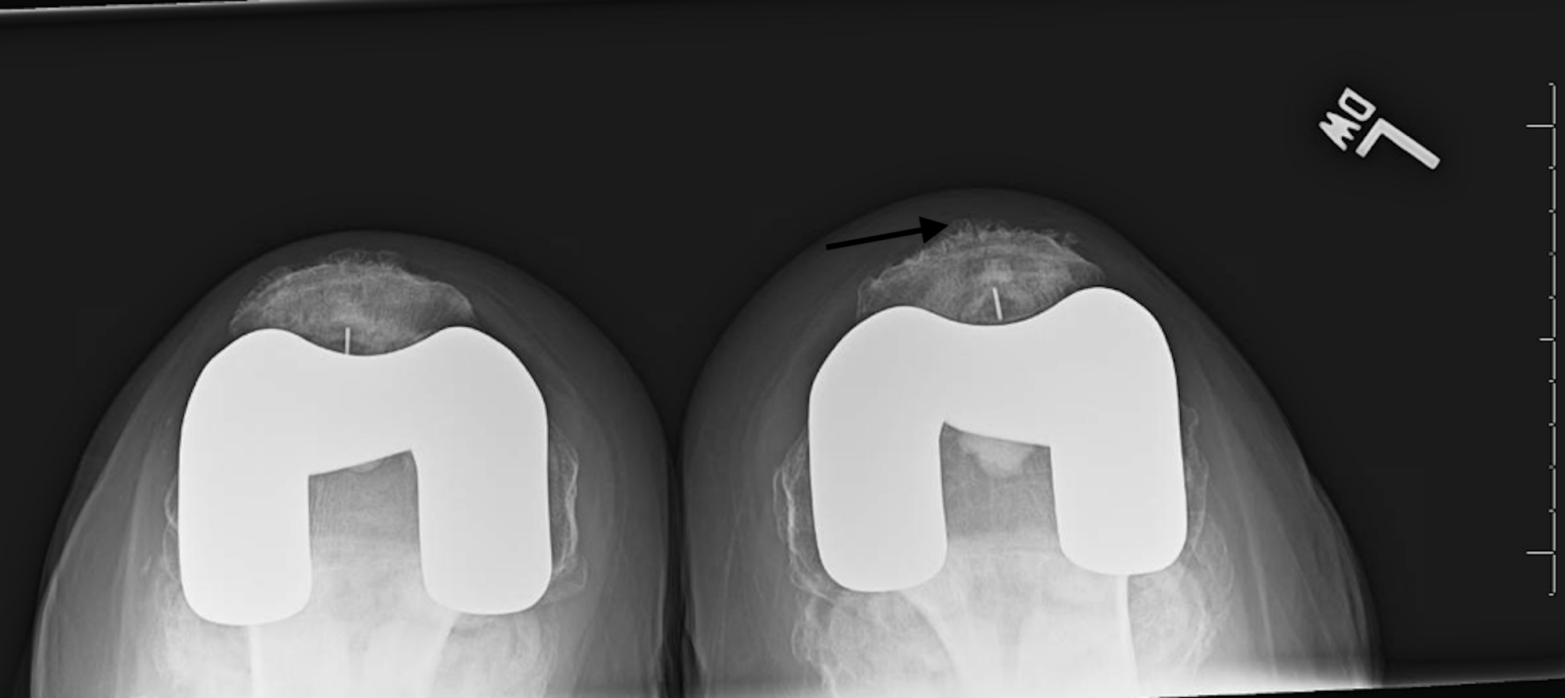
Radiograph of the case at initial presentation showing osteochondral bodies in left suprapatellar recess
